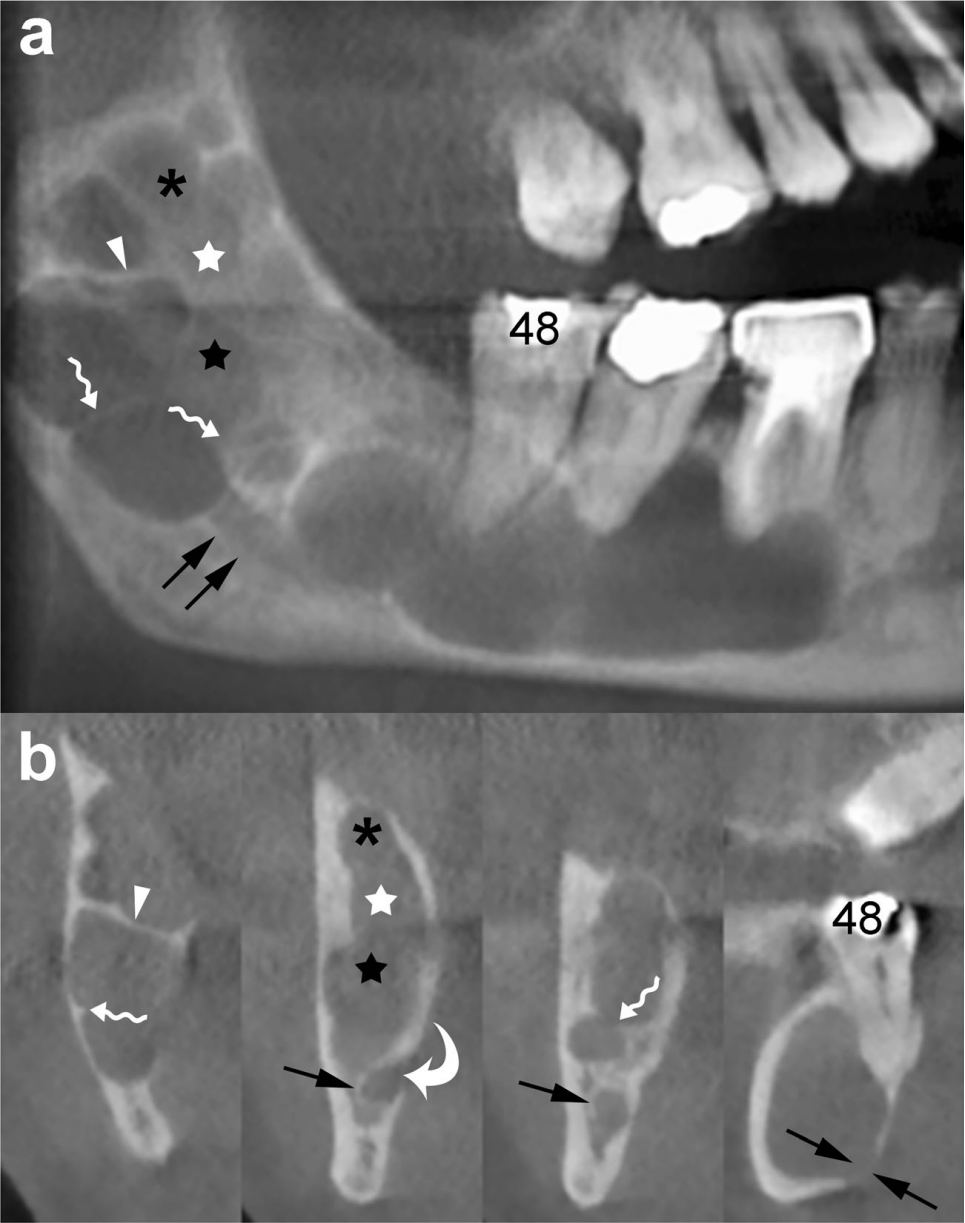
Panoramic CBCT image with 20-mm slice thickness (a) shows a multilocular OKC. On this reconstruction, septa seem to divide the lesion into multiple loculations. Cross-sectional CBCT images reconstructed as 0.5-mm-thick sections (b) demonstrate that some of these septa are complete (*arrowheads*) and some are incomplete (*wavy arrows*). Note that certain small loculations shown by the panoramic CBCT image (*black asterisk*, *white and black stars*) actually correspond to a single large loculation with scalloped borders. Compression and lingual displacement of the mandibular canal, deep to the root of the third molar (*48*), is also shown (*opposing arrows*). *Curved arrow*, mandibular foramen; *arrows*, mandibular canal
